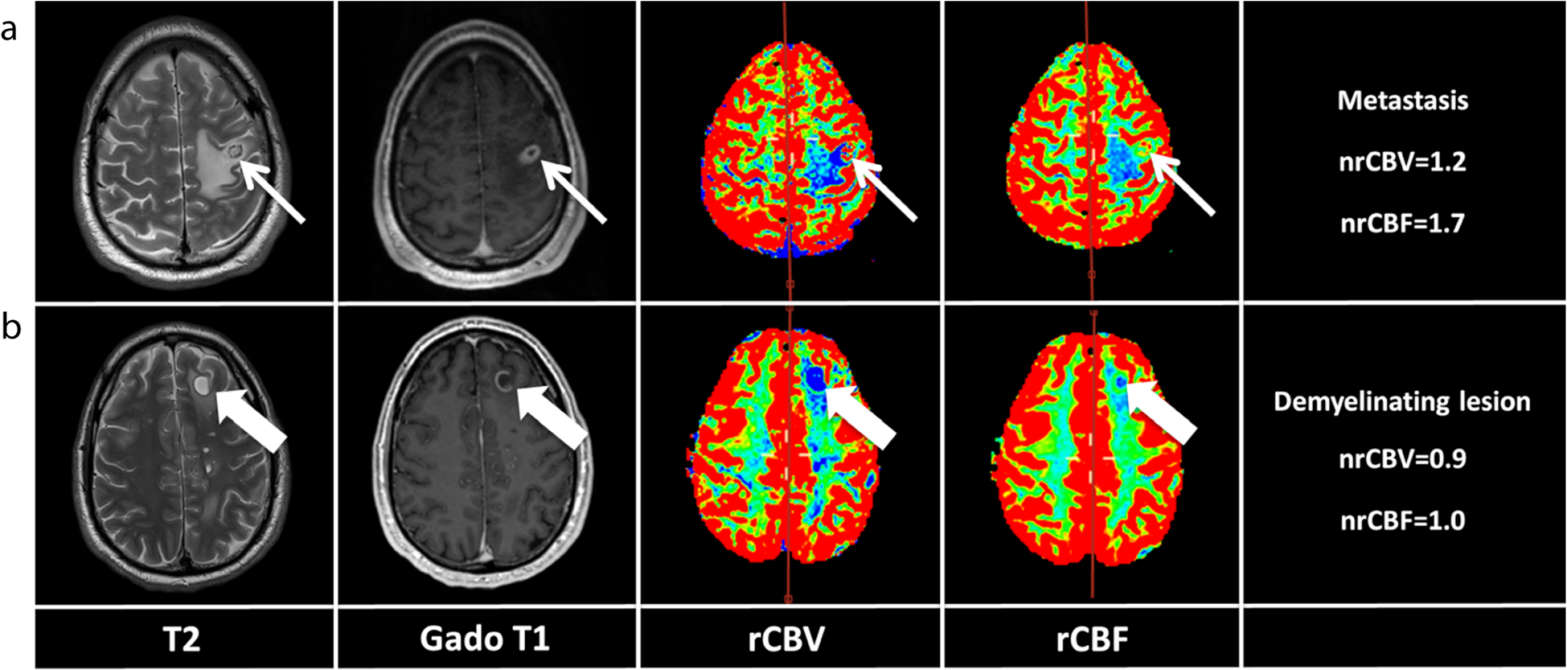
Comparison of the appearance of metastasis and demyelinating lesion in this patient. a The patient was treated by stereotaxic radiotherapy in January 2018 for several brain metastases, the largest being located at the cortico-subcortical junction of the left precentral gyrus. The lesion appeared hypointense with central necrosis and large perilesional oedema on T2-weighted images, had complete ring enhancement on post-contrast T1-weighted images, and increased normalized cerebral blood volume (nrCBV = 1.2) and cerebral blood flow (nrCBF = 1.7) ratios on perfusion weighted images. b In June 2018, he developed several demyelinating lesions, the largest being located in the left superior frontal gyrus. Contrary to the brain metastasis, the lesion was juxtacortical, had little perilesional oedema, partial ring enhancement (i.e. open ring sign), and low nrCBV and nrCBF
